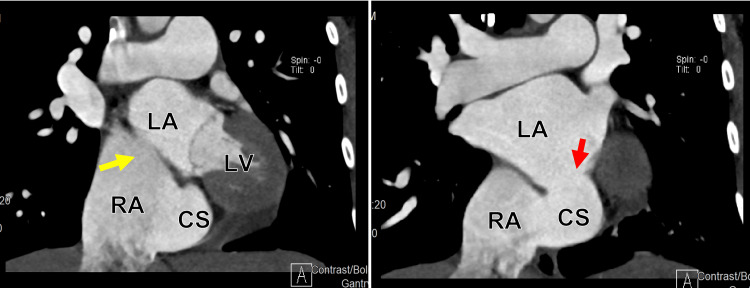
Cardiac computed tomography angiography (CCTA) in sagittal displays showing a dilated RA, an ASD (yellow arrow), and an unroofed CS (red arrow). RA: Right atrium; CS: Coronary sinus; ASD: Atrial septic defect.
